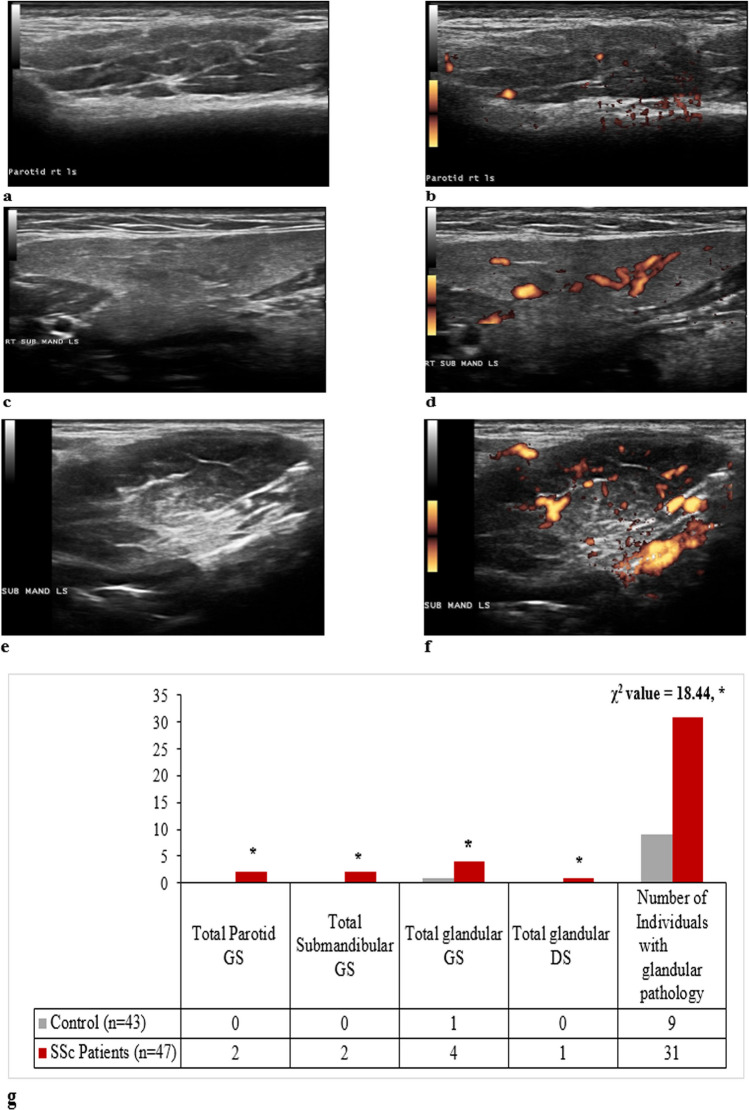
Representative ultrasound images showing four-grade semiquantitative scoring system of salivary gland. a GSUS of the parotid (grade 2). b PDUS of the parotid gland (grade 1). c GSUS changes of the submandibular gland (grade 1). d PDUS of the submandibular gland (grade 2). e GSUS of the submandibular gland (grade 3). f PDUS of the submandibular gland (grade 3). g Data are expressed as median (interquartile range) for nonparametric variables and frequencies (percentages) for categorical variables. SSc, systemic sclerosis; GS, gray scale; DS, Doppler signal; GSUS, gray scale ultrasound; PDUS, power Doppler ultrasound. A single asterisk (*) denotes significance at *P* < 0.0001, compared to the control individuals. The mean difference is significant at *p* < 0.05 using asymptotic significance 2-sided
